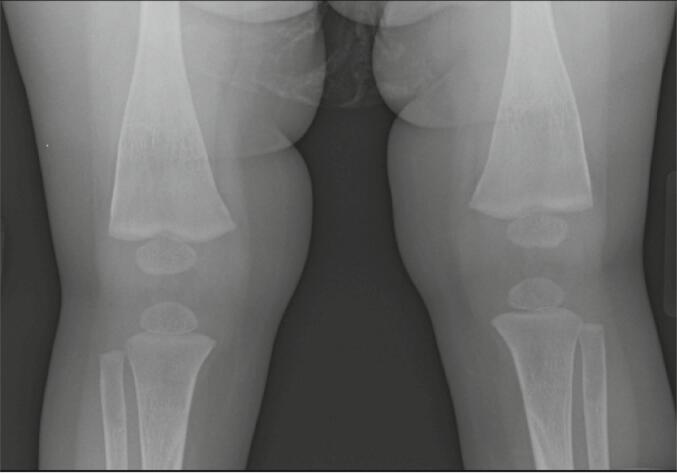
Radiographic study of the knees at 22 months of age, showing resolution of rachitic findings in distal femurs.
